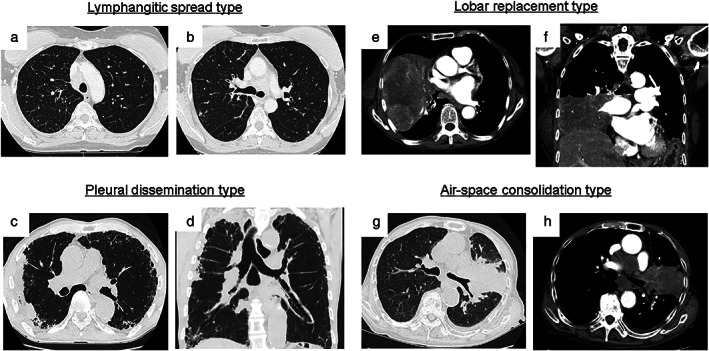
Representative images of the tumor extension and spread pattern of atypical types of small‐cell lung cancer. Typical thin‐sliced computed tomography images of the lymphangitic spread type (a and b), pleural dissemination type (c and d), lobar replacement type (e and f), and air‐space consolidation type (g and h)
